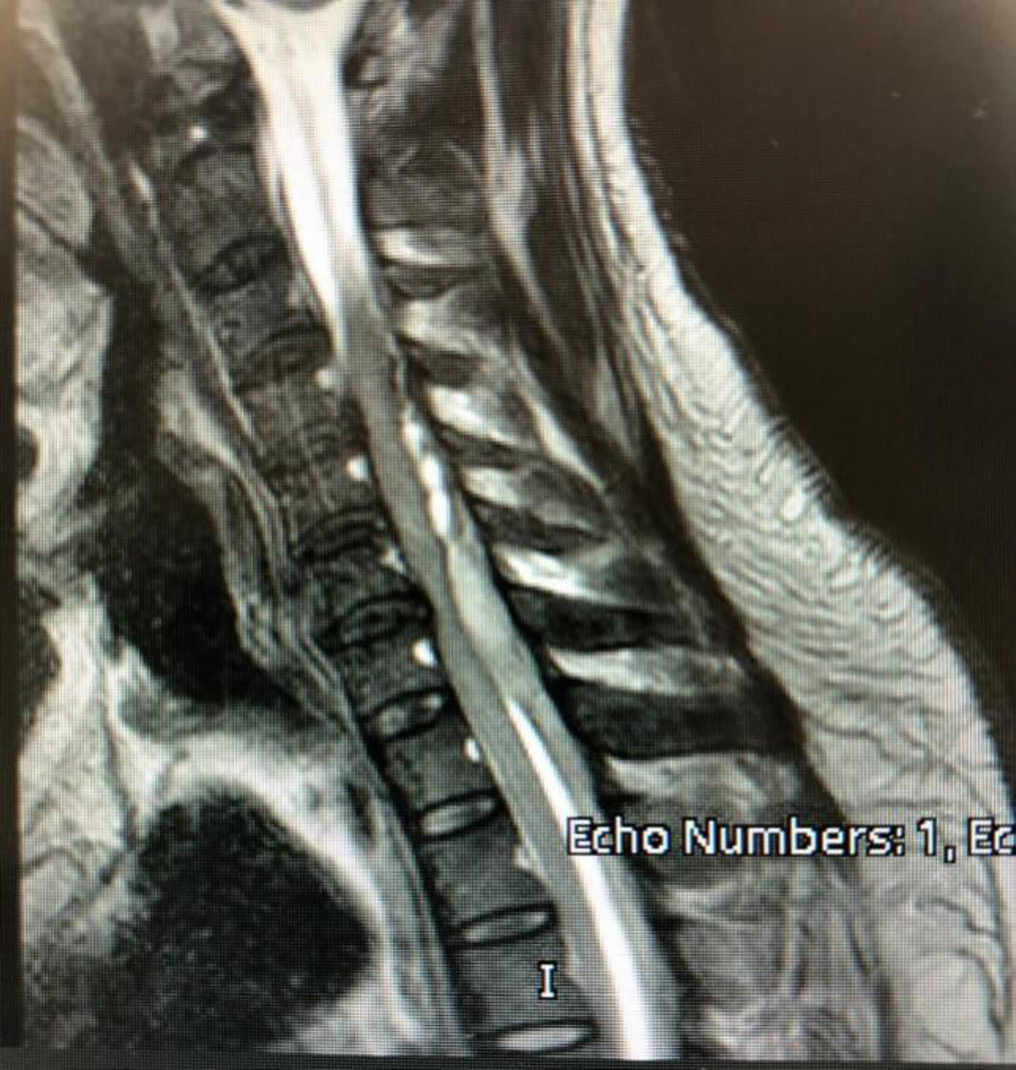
MRI showing a posterior extradural collection.
